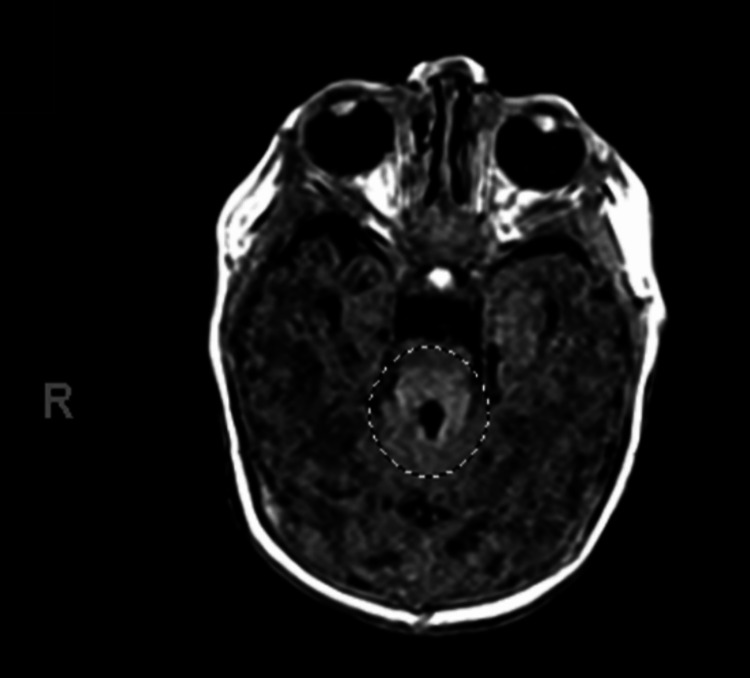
MRI of the brain showing relative hypoplasia of portions of the midbrain with a molar tooth sign as indicated by the circle
